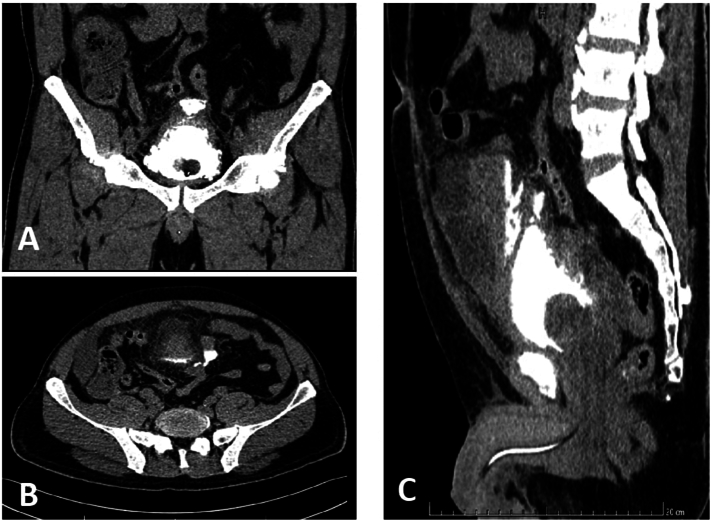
Abdominopelvic CT scan demonstrating contrast extravasation, a: Coronal view, B: Axial view, C sagittal view.
